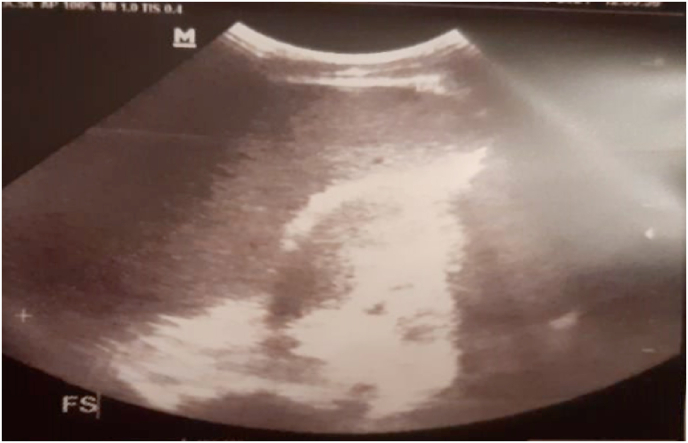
Abdominal ultrasound: showing splenomegaly measuring 14 cm.
